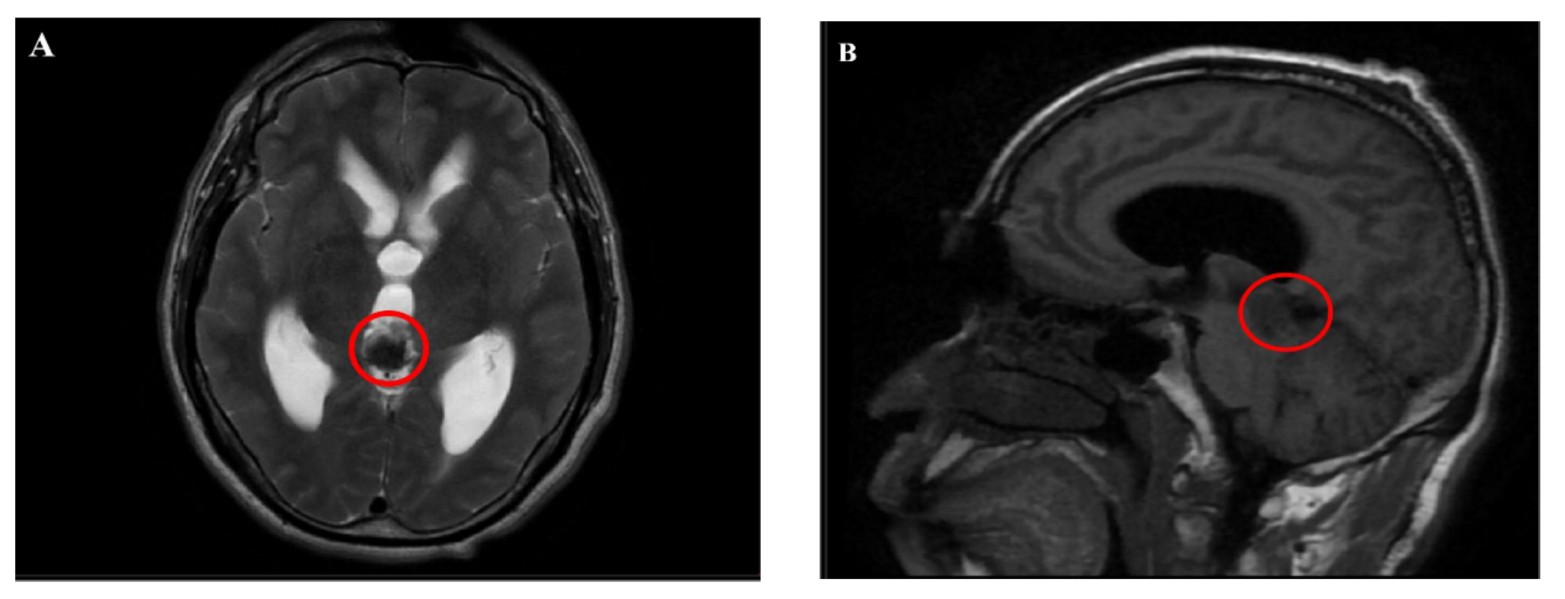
(A, B) MRI scanning revealed an abnormal signal nodular shadow in the pineal region.
